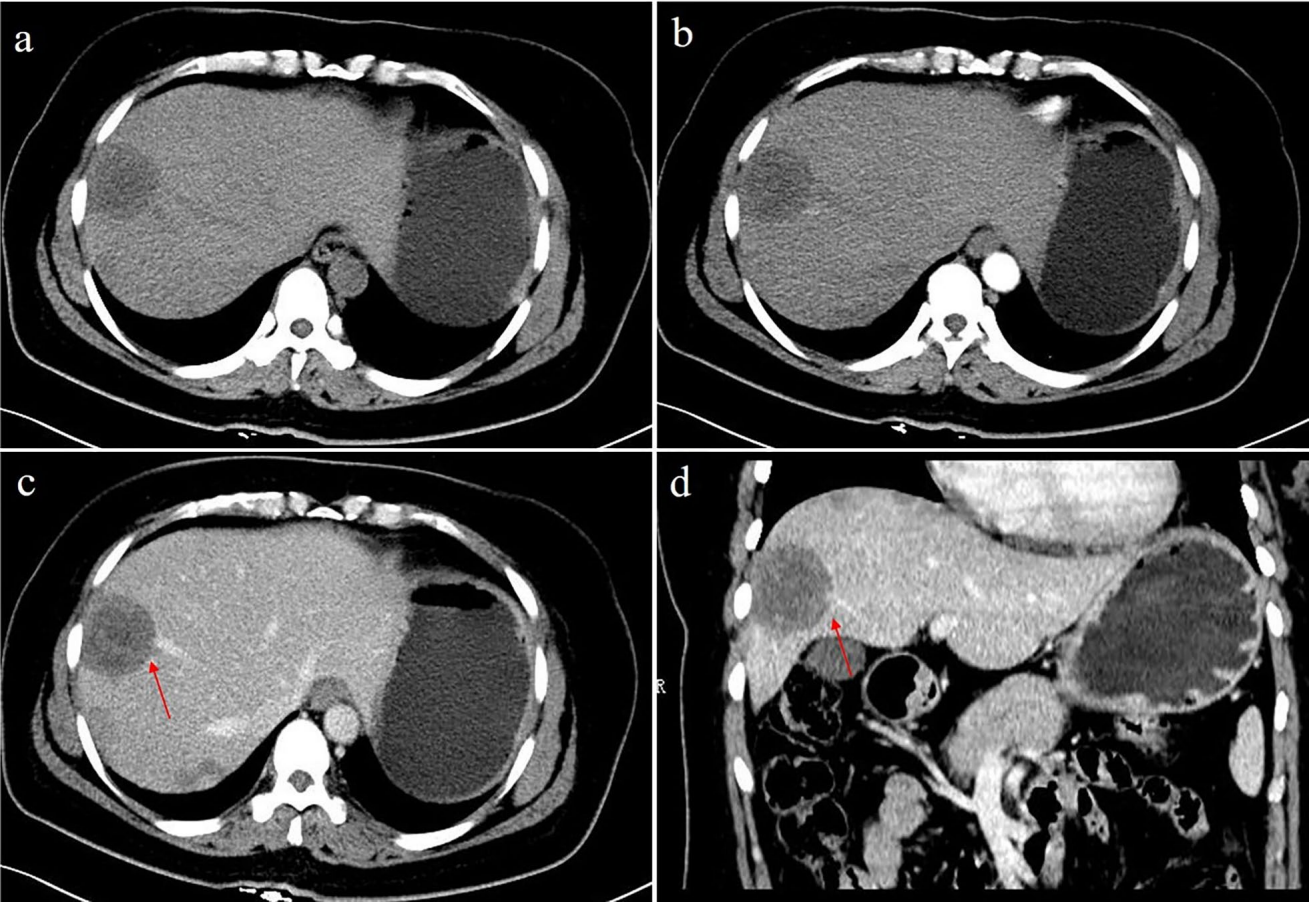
A 48-year-old female with multifocal nodular type HEH. An axial unenhanced CT image (a) shows multiple round-like low-density lesions in the right lobe of the liver. The lesions show slight enhancement in the arterial phase (b). Axial and coronal reconstruction of portal phase CT images (c, d) show a larger nodule in the right lobe with portal veins entering and terminating in the periphery of the lesion (red arrow). This configuration resembles a typical “lollipop” sign
